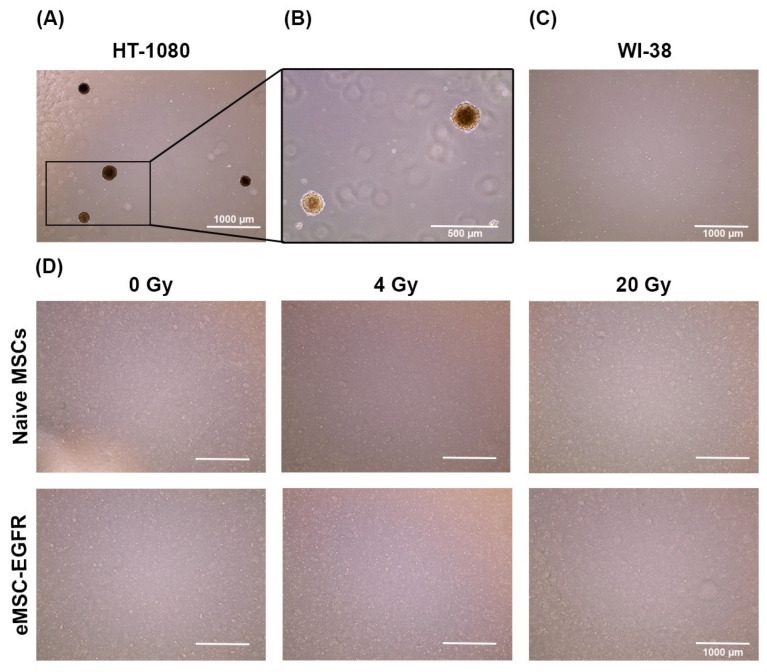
Tumorigenicity assay of naïve and engineered MSCs with EGFR after single-dose X-ray exposure. Cells were plated in soft agar to assess their ability to form colonies, indicative of tumorigenic potential. (A,B) 5000 cells/well of positive control cell line HT-1080 and (C) 67,000 cells/well of WI-38 negative control cell line were used as controls to validate the procedure. (D) 67,000 cells/well of naïve MSCs and eMSC-EGFR were cultured for three weeks to assess tumorigenic potential 24 h following no X-ray exposure (0 Gy), 4 Gy and 20 Gy (A,C,D) scale bar: 1000 µm (B) scale bar: 500 µm.
